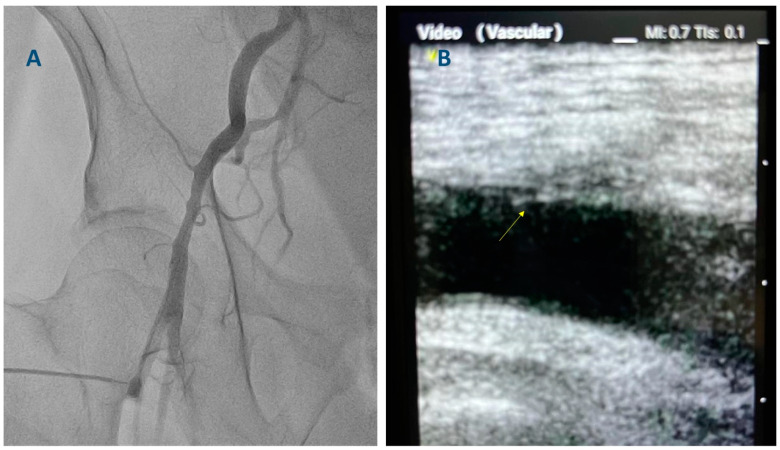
(A) Femoral angiography of a patient who will undergo coronary angiography. (B) Right femoral imaging with ultrasound in the same patient after coronary angiography completion (longitudinal axis). An Angio-Seal vascular closure device was placed in the right femoral artery (arrow).
